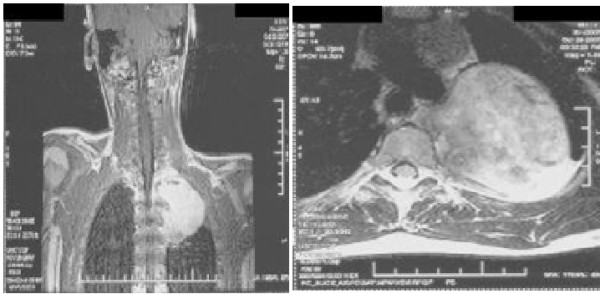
MRI scan showing homogenous soft tissue density mass in the posterior mediastinum. (a) Sagittal section. (b) Axial section.
